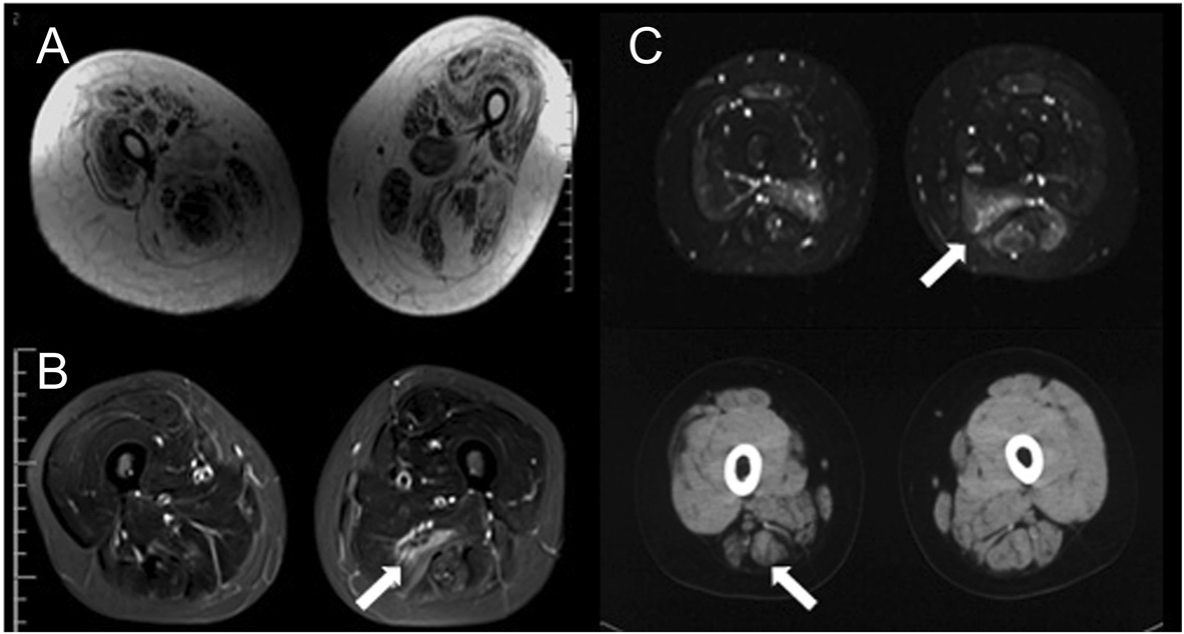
Muscle images of thighs in patients with anti-SRP antibodies. (a) Muscle atrophy on MRI T1 images. (b) High signal intensity on MRI STIR images. (c) Increased signals on MRI STIR images (upper) and muscle atrophy in CT (lower)
